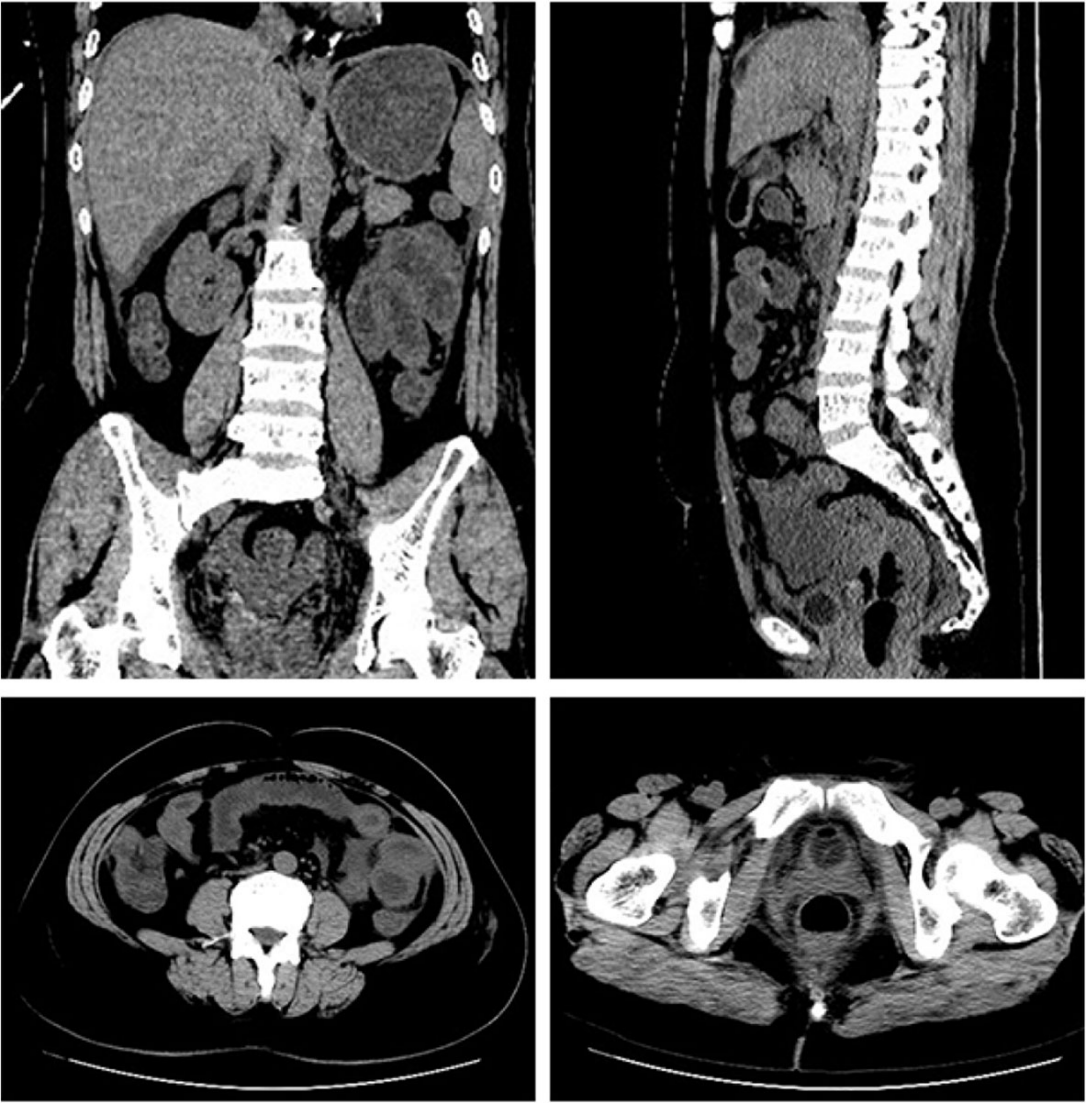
CT plain scan of the pelvic and abdominal cavity
